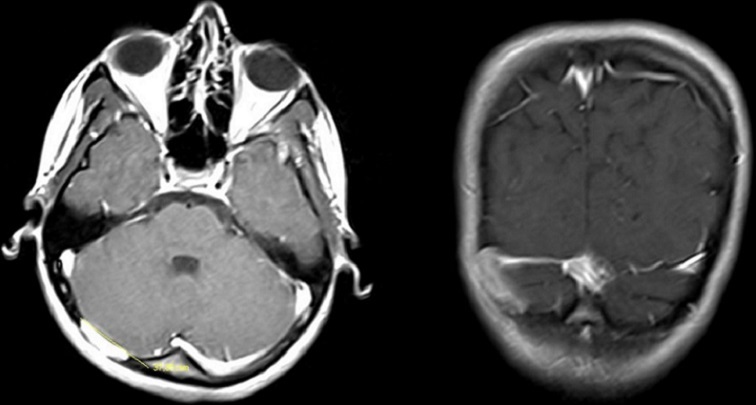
Gadolium enhancing cranial MR image of bone defect and surrounding lesion with high gadolinium enhancement
